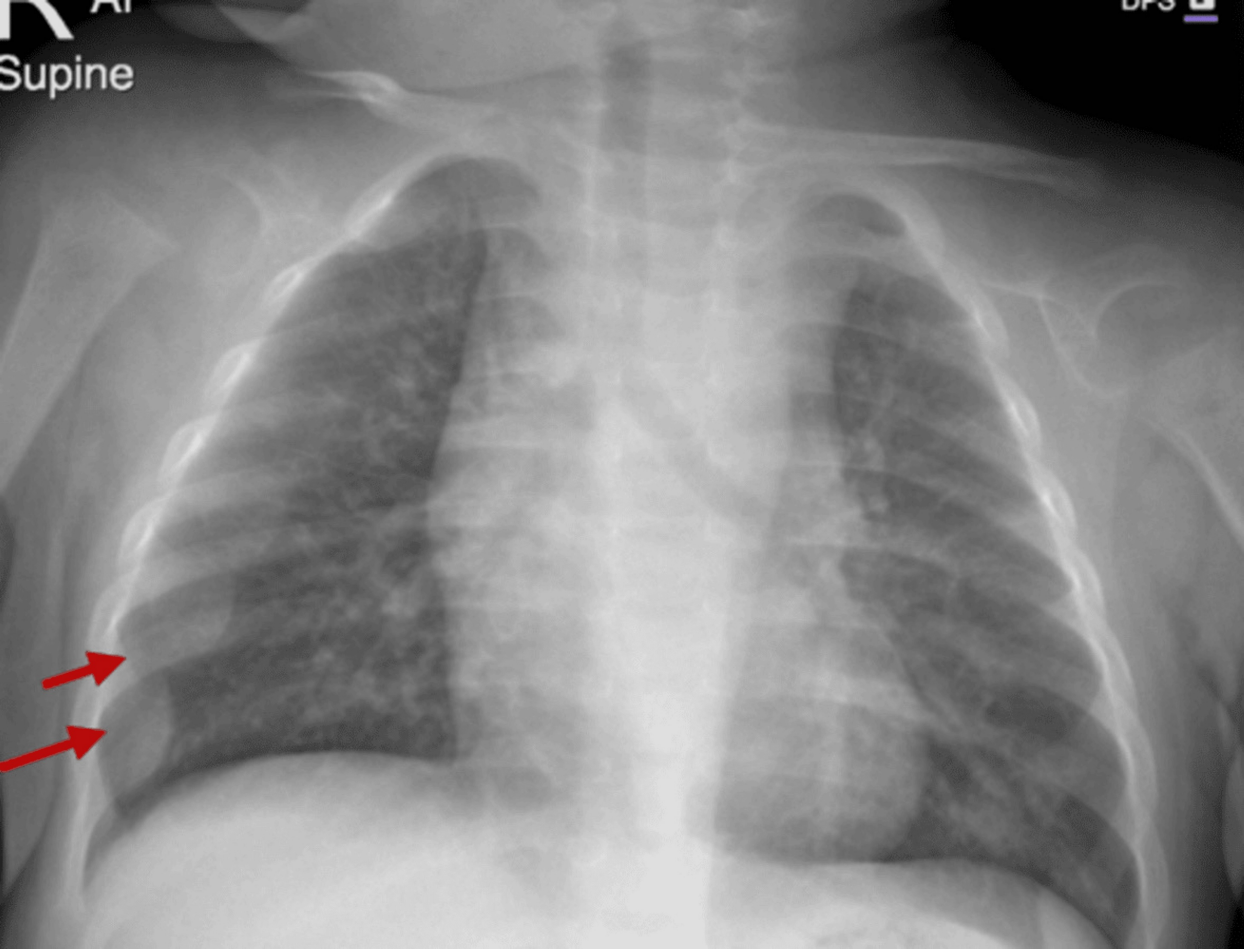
Chest X-ray showing mild rachitic rosary (arrows) and osteopenia
